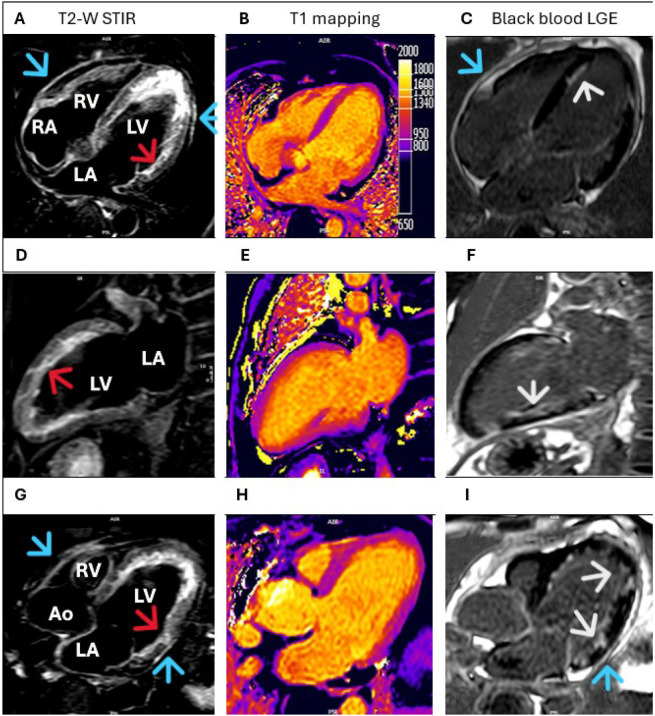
To examine the cause of myocardial injury, cardiac magnetic resonance imaging (CMR) was performed. STIR sequences (panels (A,D,G), red arrows) showed subendocardial and transmural myocardial hyperintensity, indicating edema. T1-mapping sequences (panels (B,E,H)) revealed increased native myocardial T1 values, represented on the color scale. Black blood late gadolinium enhancement (LGE) sequences (panels (C,F,I)), which improve contrast between the ventricular cavity and endocardium, demonstrated diffuse endocardial hyperintensity (white arrows), suggesting non-ischemic fibrosis. Additionally, pericardial hyperintensity was noted in the STIR (panels (A,D,G), blue arrows) and LGE (panels (C,I), blue arrows) sequences, indicating pericardial inflammation. These findings, support a diagnosis of eosinophilic myocarditis with acute pericarditis.
